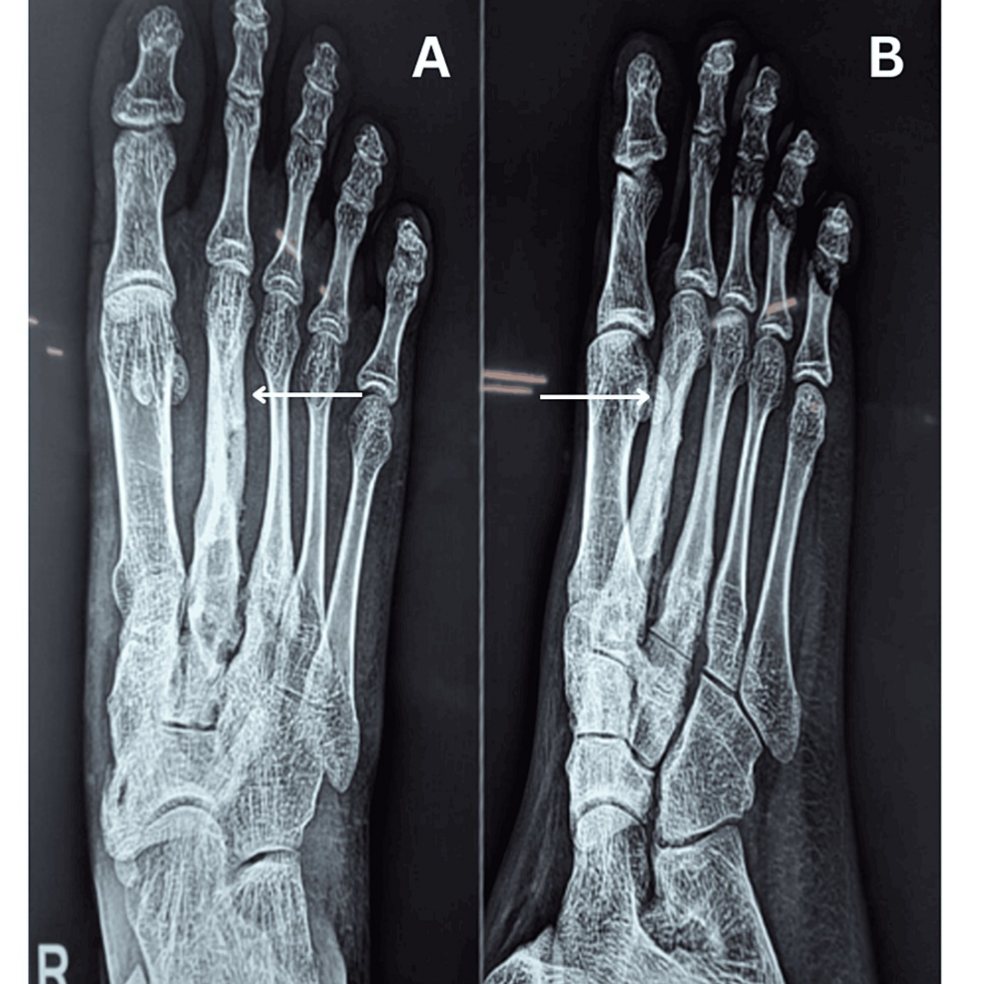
X-rays of the right foot before debridement of the second metatarsal: A) ankle AP view and B) ankle lateral view AP: anteroposterior
